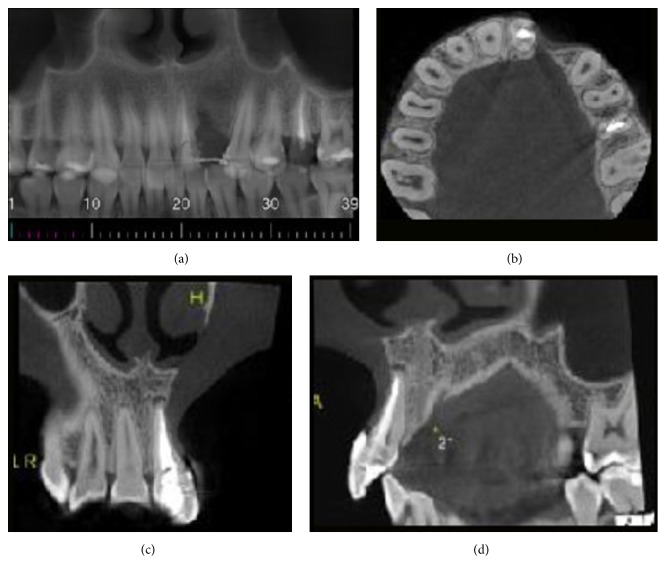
(a) CT panoramic reconstruction of maxilla. (b) CT axial reconstruction of the jaw. (c) CT coronal reconstruction of anterior region of maxilla. (d) CT sagittal reconstruction of tooth 11 region.
